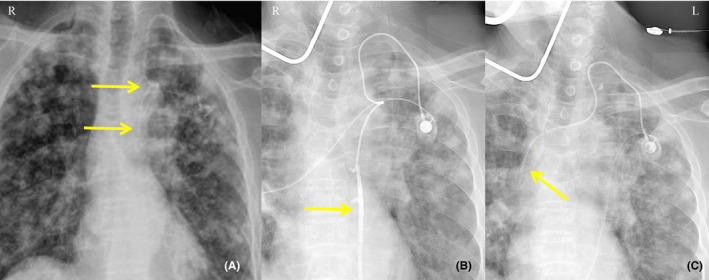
X‐ray radiography and DSA findings in Case 2. A, Postoperative X‐ray radiographic examination revealed abnormal positioning of the catheter tip. B, DSA revealed entry of the catheter tip into the left ITV. C, Correct positioning of the catheter tip at the level of the 6th thoracic vertebra after catheter repositioning as confirmed by DSA
